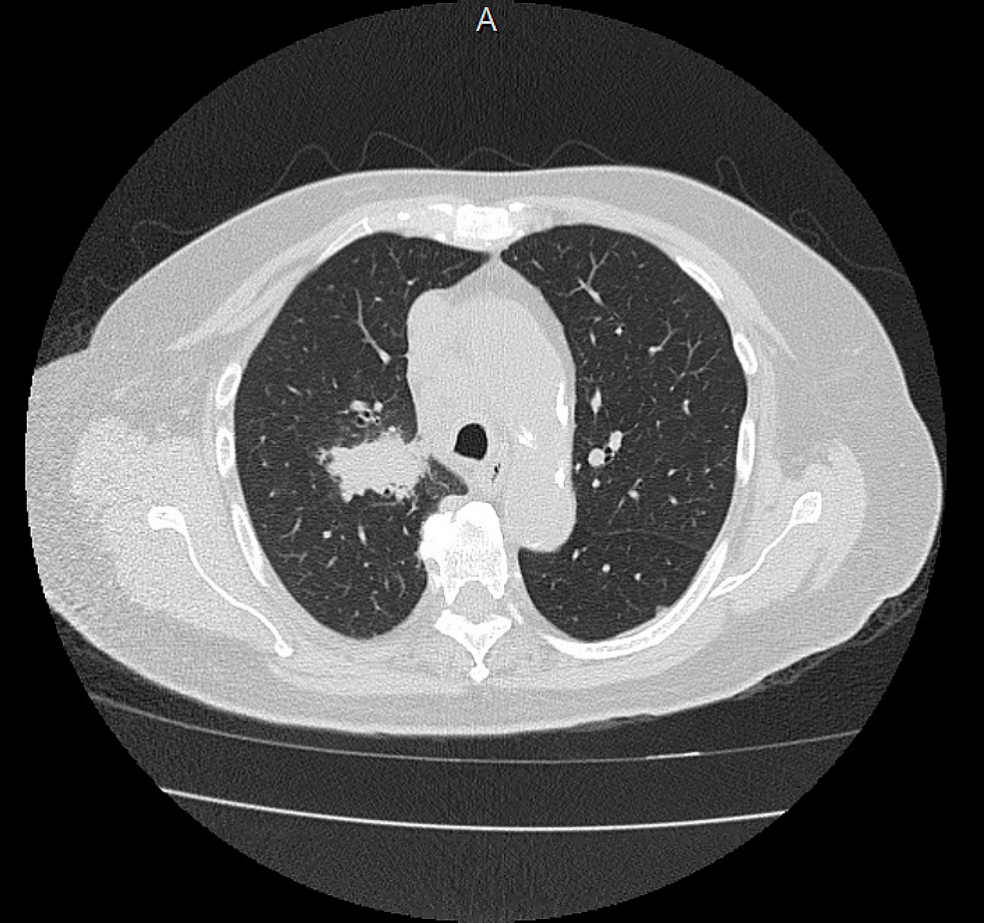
Cross-sectional CT Chest of Right Upper Lobe Opacity
